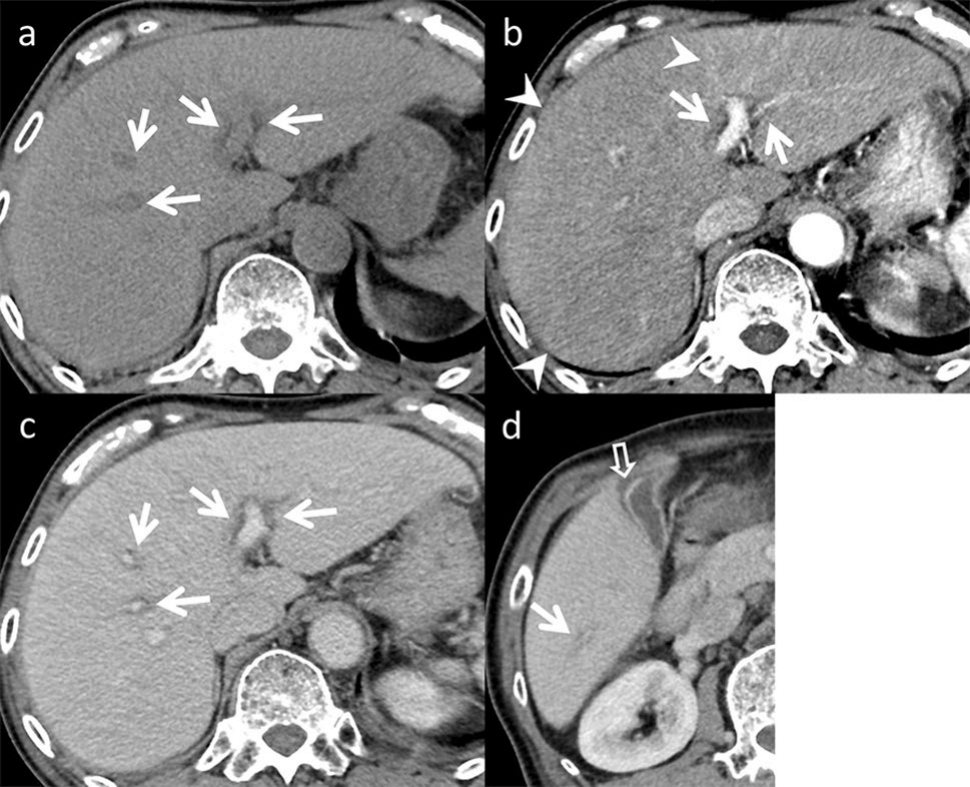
Drug-induced acute hepatitis in an 81-year-old man with appetite loss and hepatobiliary enzyme elevation, who was taking tizanidine for 1 month. Dynamic computed tomography (CT) (a pre-contrast, b arterial phase, and c portal venous phases) demonstrates periportal edema (arrows). b The liver parenchyma is heterogeneously enhanced in the arterial phase (arrowheads). d The gallbladder wall is thickened in the portal venous phase (open arrow). However, the gallbladder is not enlarged or distended, differentiating it from acute cholecystitis. The hepatobiliary enzyme levels normalized after tizanidine withdrawal. On imaging, acute hepatitis from other causes is differential; however, both are nonspecific and difficult to distinguish on imaging alone
